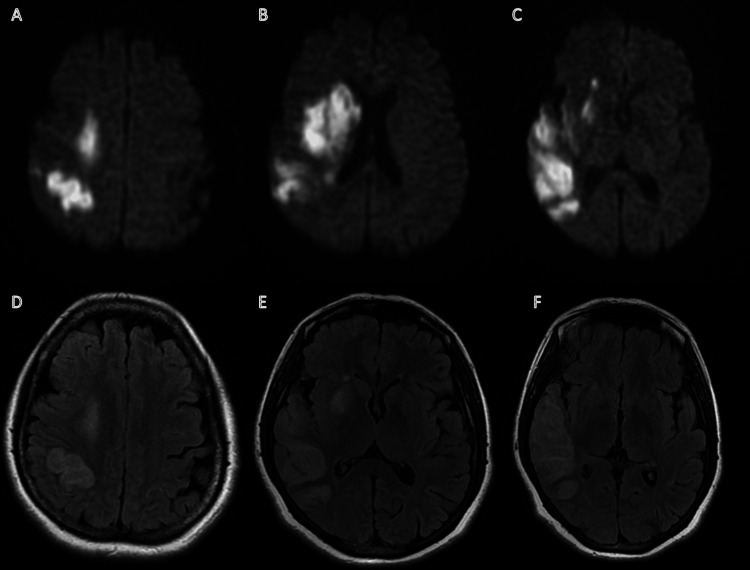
MRI study from patient 1 Panels A-B-C: DWI sequences showing multiple hyperintense foci in the right primary motor cortex (A), in the deep gray and white matter of the frontal lobe (B) and in the temporal lobe (C). Panels D-E-F: FLAIR sequences showing the corresponding ischemic areas.
